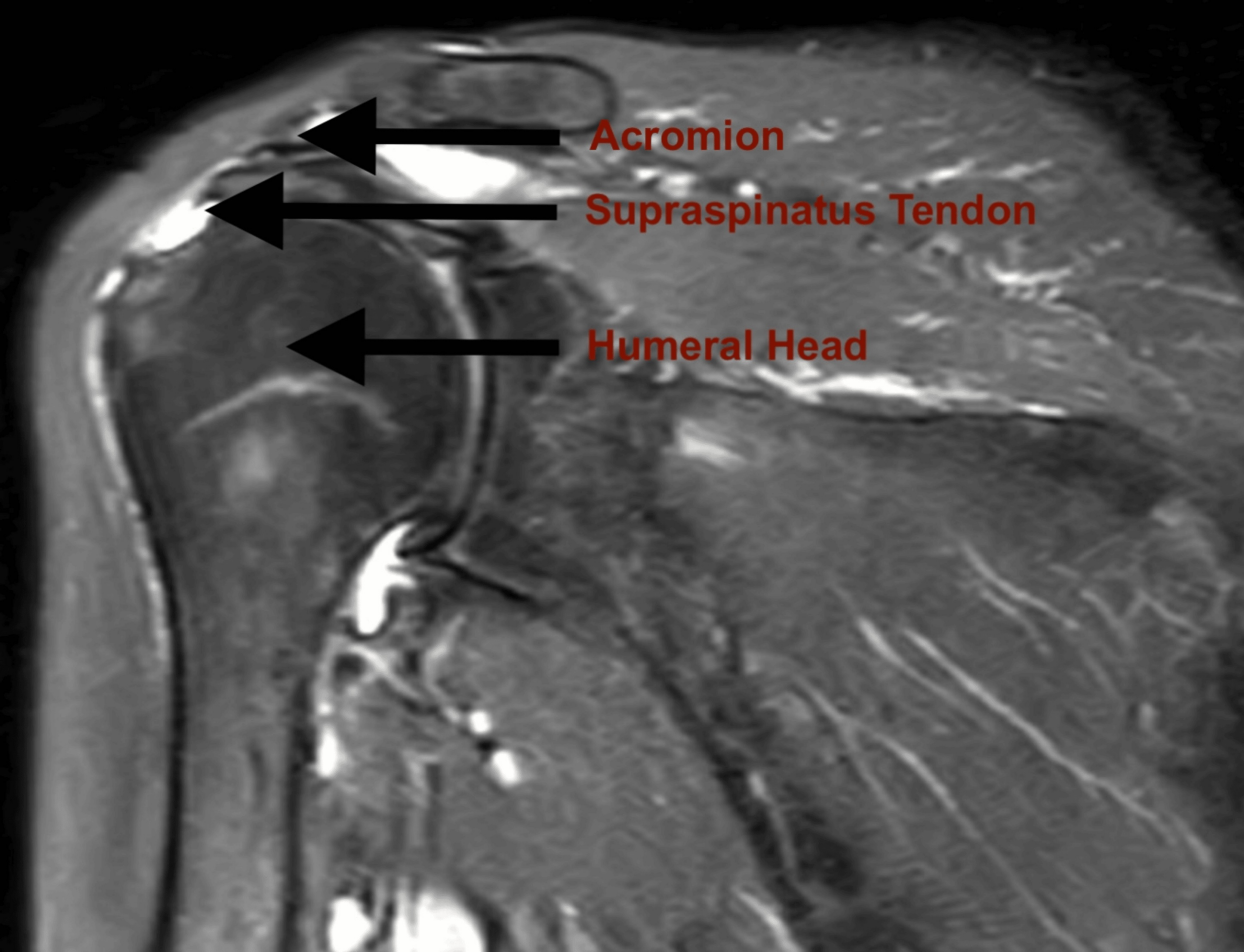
Patte classification stage 2 shoulder magnetic resonance imaging
